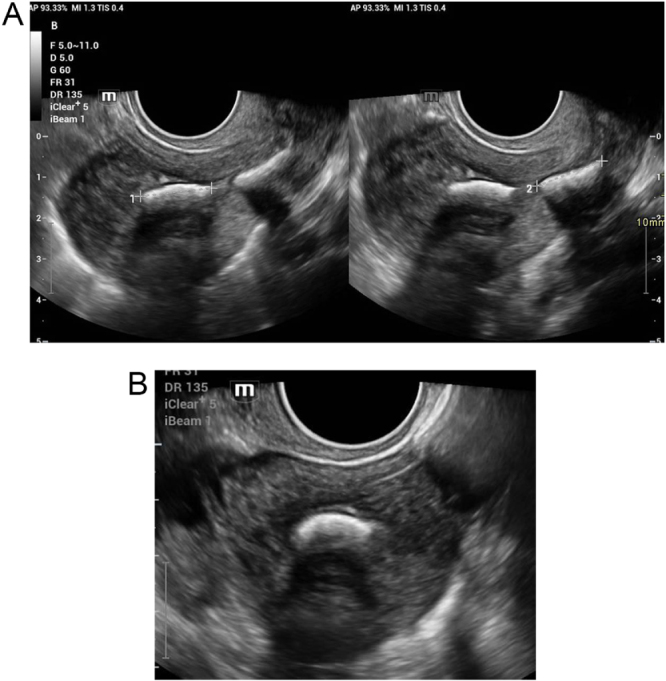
(A). Transvaginal ultrasound sagittal views demonstrating linear hyperechoic intrauterine structures, suggestive of retained fetal bones/endometrial calcifications prior to hysteroscopic confirmation. (B). Transvaginal ultrasound transverse views demonstrating linear hyperechoic intrauterine structures, suggestive of retained fetal bones/endometrial calcifications prior to hysteroscopic confirmation.
